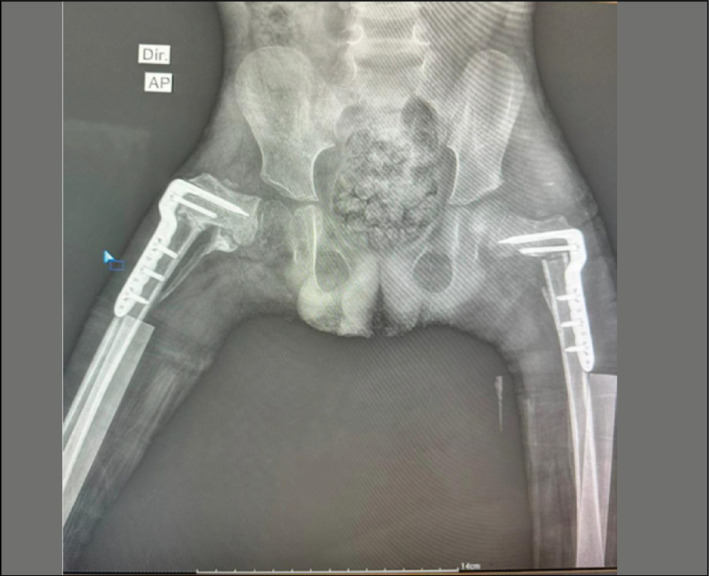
Postoperative follow-up radiograph of a child with cerebral palsy who underwent bilateral varus derotational femoral osteotomy.
